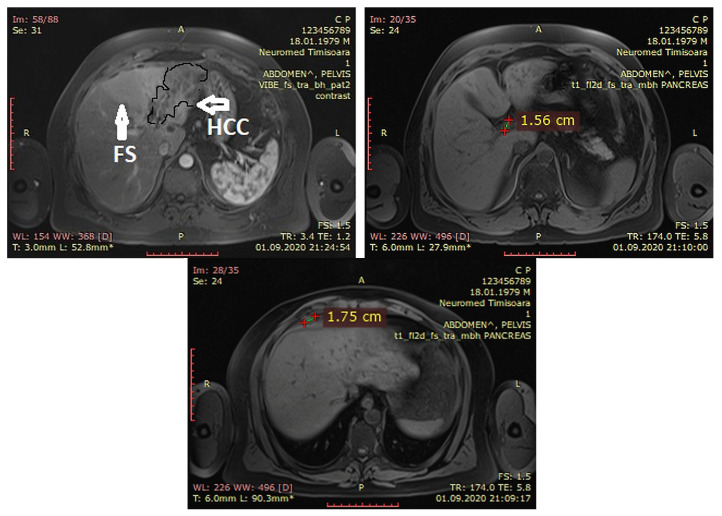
Dynamic contrast-enhanced abdominal MRI. (Left): Focal steatosis (vertical arrow) and liver mass of the left lobe (horizontal arrow). (Right): Secondary nodules at the segment 1 of the liver. (Middle): Secondary nodules at the right supra-diaphragmatic fat. (FS = focal steatosis, HCC = hepatocellular carcinoma).
